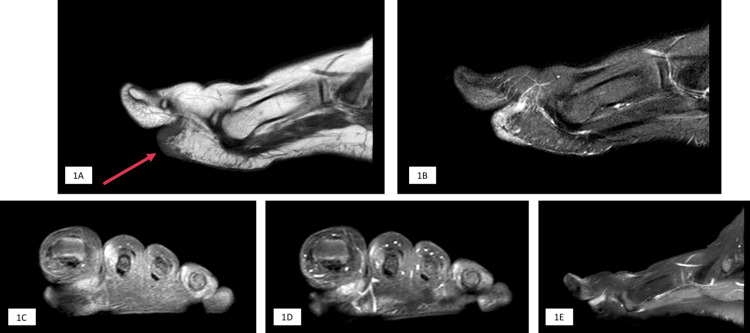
MRI sequences of the left foot (A) Sagittal T1-weighted image (B) Sagittal T2-weighted Short tau inversion recovery (STIR) image (C) Coronal T1-weighted fat-suppressed image (D) Coronal post-contrast T1-weighted fat-suppressed image (E) Sagittal post-contrast T1-weighted fat-suppressed image. Magnetic resonance images show soft tissue thickening and swelling involving dermal and subcutaneous tissue at plantar aspect of the big toe at level of proximal phalanx measure approx. 0.5 cm thickness and 2 cm length showing mild post contrast enhancement. Underlying soft tissue, tendon and bone appears intact.
